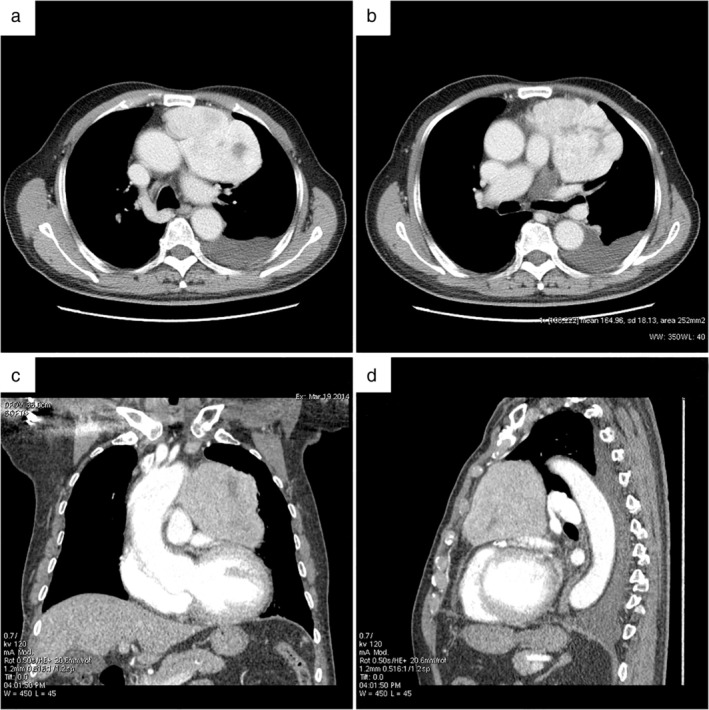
Chest computed tomography (CT) scan showed a significantly enhanced irregular huge soft tissue mass in the anterior mediastinal area (a–d).
